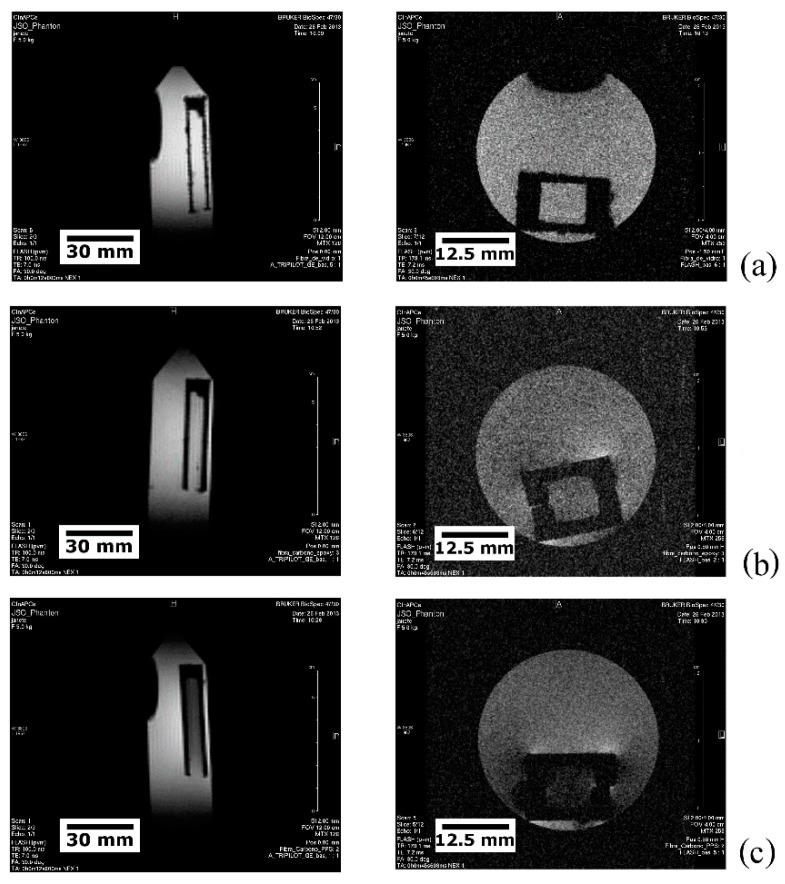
MRI tomographic views of: (a) the EPX-GF; (b) the EPX-CF; and (c) the PPS-CF vessels immersed in WSS.
